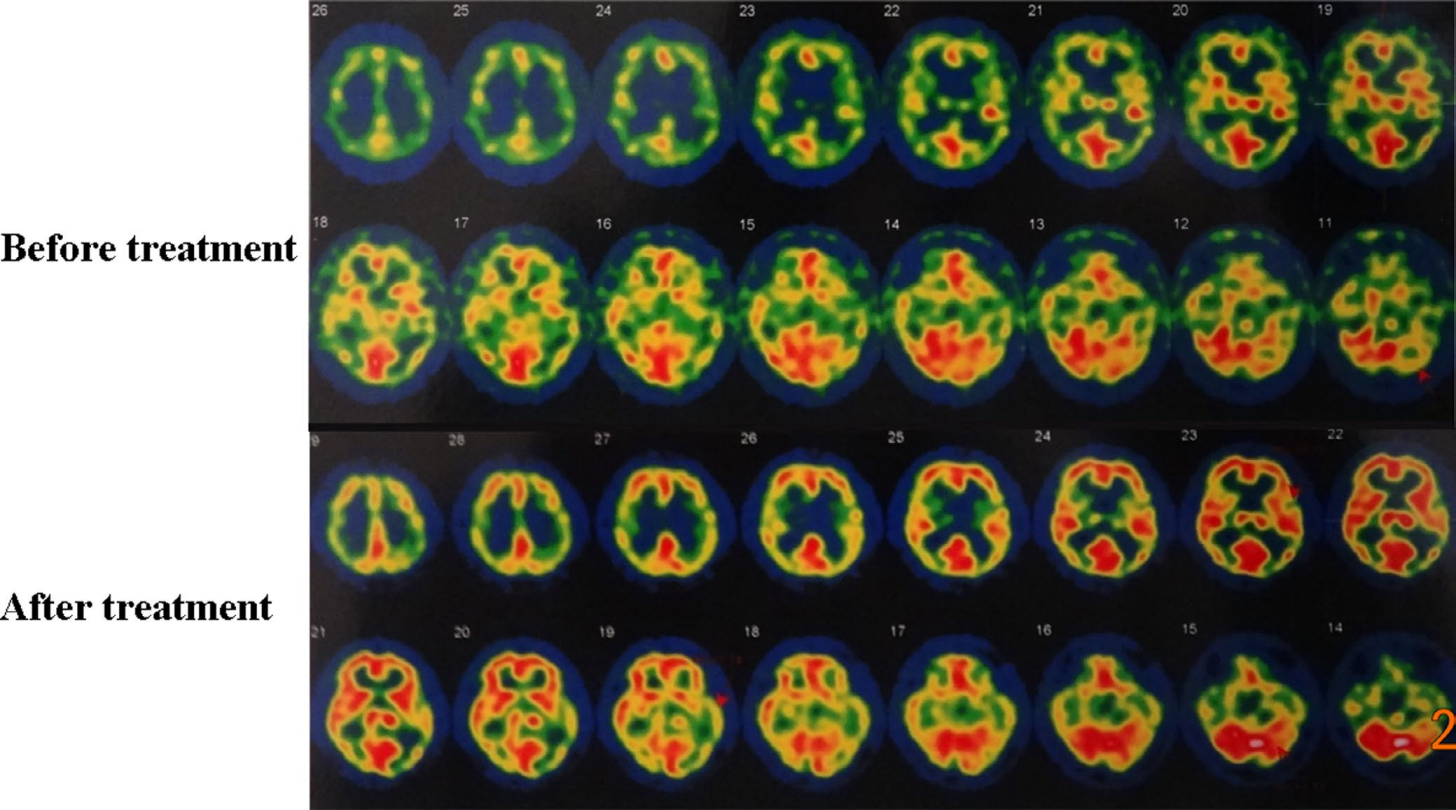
Perfusion presented on SPECT maps prior to and post‐RIC treatment. SPECT shows that the perfusion decreases in bilateral frontal, parietal, occipital, and temporal lobes before RIC regimen. After 1 year of RIC treatment, the perfusion is significantly enhanced in all lobes
